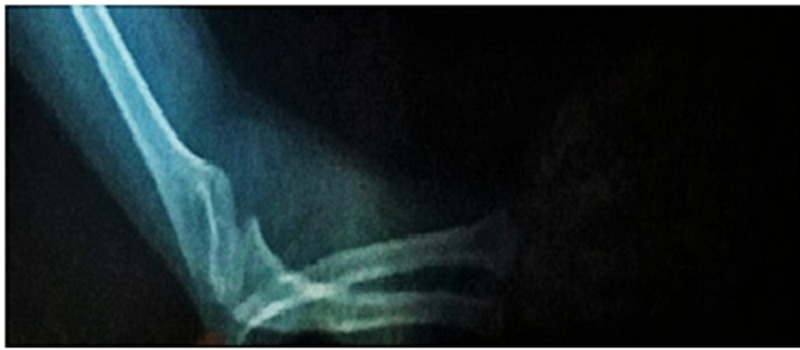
Case one: evidence of posterior dislocation of the radial head as seen in the lateral view of the right elbow X-ray Image quality turned out to be poor as the child was agitated and irritable, making radiographic imaging extremely difficult
